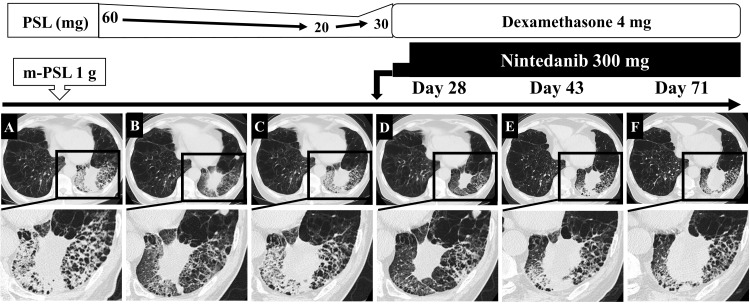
The clinical course and HRCT findings. Magnified views (bottom) of the areas enclosed by squares (top) are shown. (A) HRCT images showing the initial diagnosis of RILI, and GGOs, reticular shadows, and fibrotic changes were detected in the left lower lung of the irradiated area 8.5 months after SBRT. (B) After methyl-prednisolone pulse therapy, the GGOs with the RILI area were reduced. (C) After steroid tapering, at a dose of 20 mg/day, RILI with fibrosis exacerbated and became resistant to steroid therapy. (D) HRCT showed improvement in GGO 28 days after combination therapy of nintedanib (300 mg/day) with dexamethasone (4 mg/day). (E, F) The areas of GGO and consolidation gradually increased again. M, months; HRCT, high-resolution computed tomography; RILI, radiation-induced lung injury; GGOs, ground-glass opacities; SBRT, stereotactic body radiation therapy; m-PSL, methyl-prednisolone; PSL, prednisolone.
